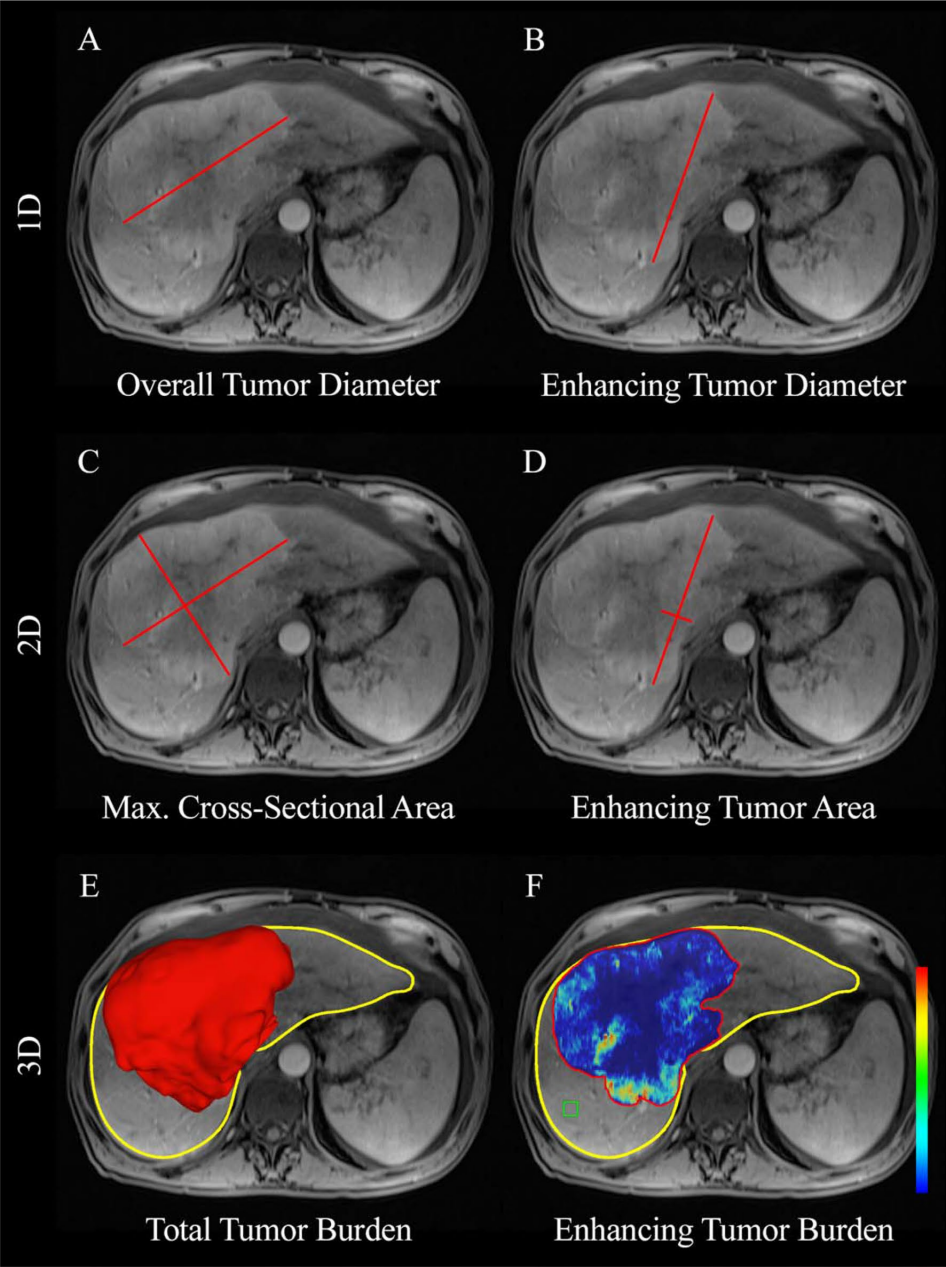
Methods of tumor assessment on enhancement-based MRI. (A,B) Demonstrate 1D measurements showing the largest overall tumor diameter (cm) and largest enhancing tumor diameter (cm) represented by the red line. (C,D) Show the 2D tumor measurement methods and represent the maximum cross-sectional area (cm^2^) and maximum enhancing tumor area (cm^2^). (E) Segmentation of the tumor (3D reconstruction in red) showing the total tumor volume (cm^3^) in relation to the total liver volume (cm^3^), shown by the yellow outline. (F) Assessing enhancing tumor volume (cm^3^). Dark-blue regions of the color map represent necrotic tissue; red regions show viable tumor tissue, as described in previous literature^19^. The region of interest (ROI) represented by the green 1 cm^3^ box is used as the relative baseline enhancement of healthy liver parenchyma to calculate the differential enhancement within the segmentation-mask of the tumor.
